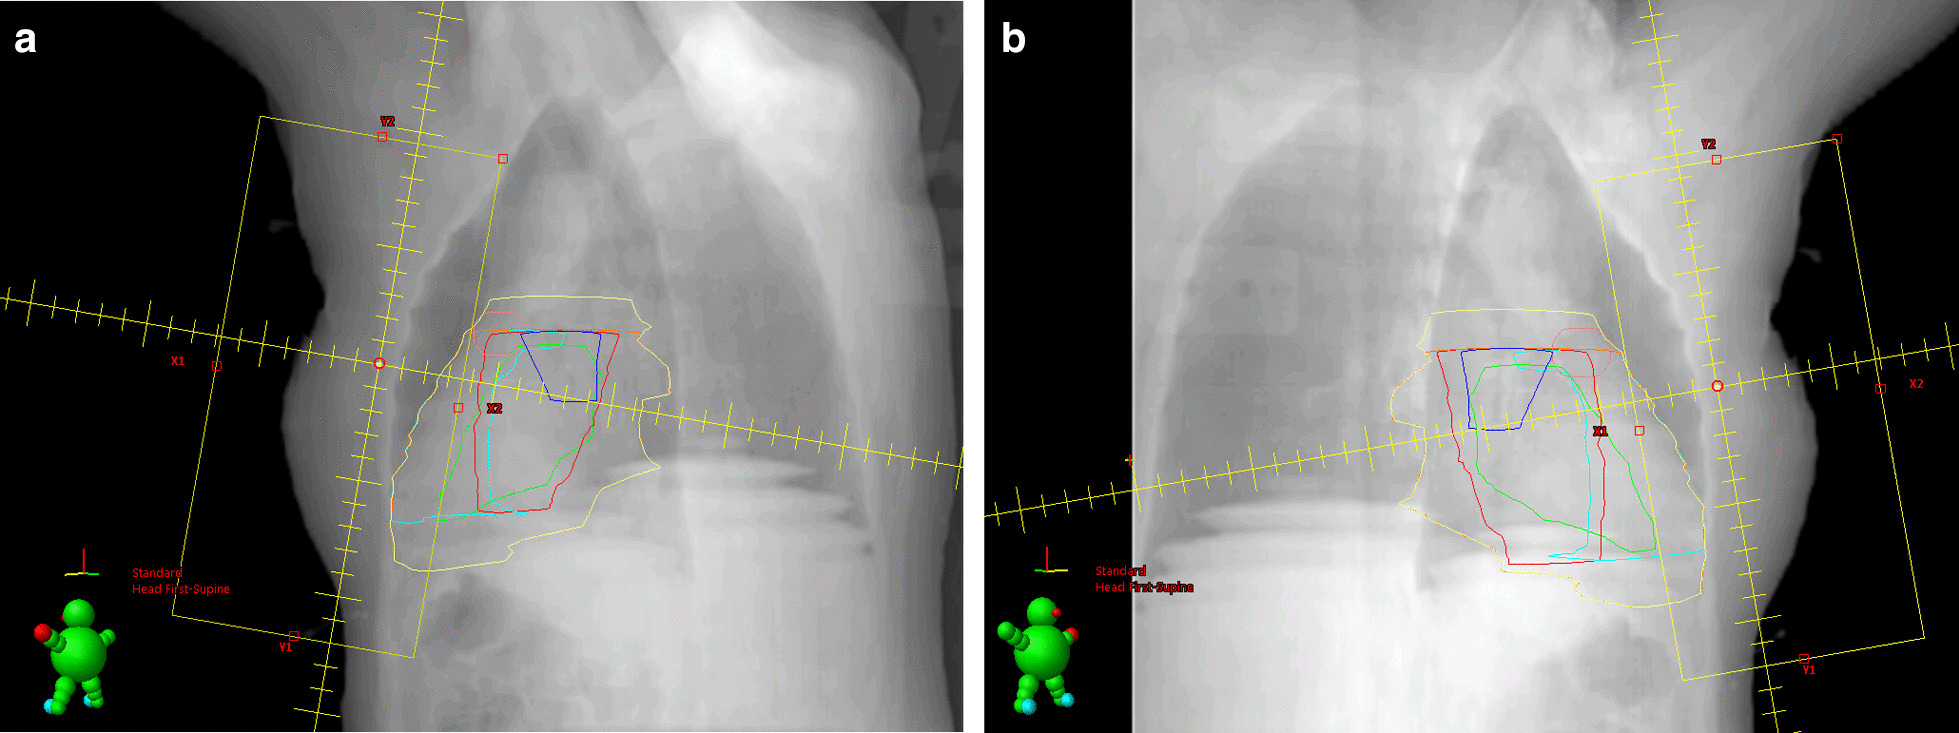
Heart atlas. Sagittal CT slices (a: patient facing left, b: patient facing right) with superimposed contours of the complete heart (orange) and functional substructures as defined by the study protocol: Aortic valve (light pink), Pulmonary valve (blue), deep structures (light green), right anterior myocardium (light blue), left anterior myocardium (red), and complete myocardium (purple region)
